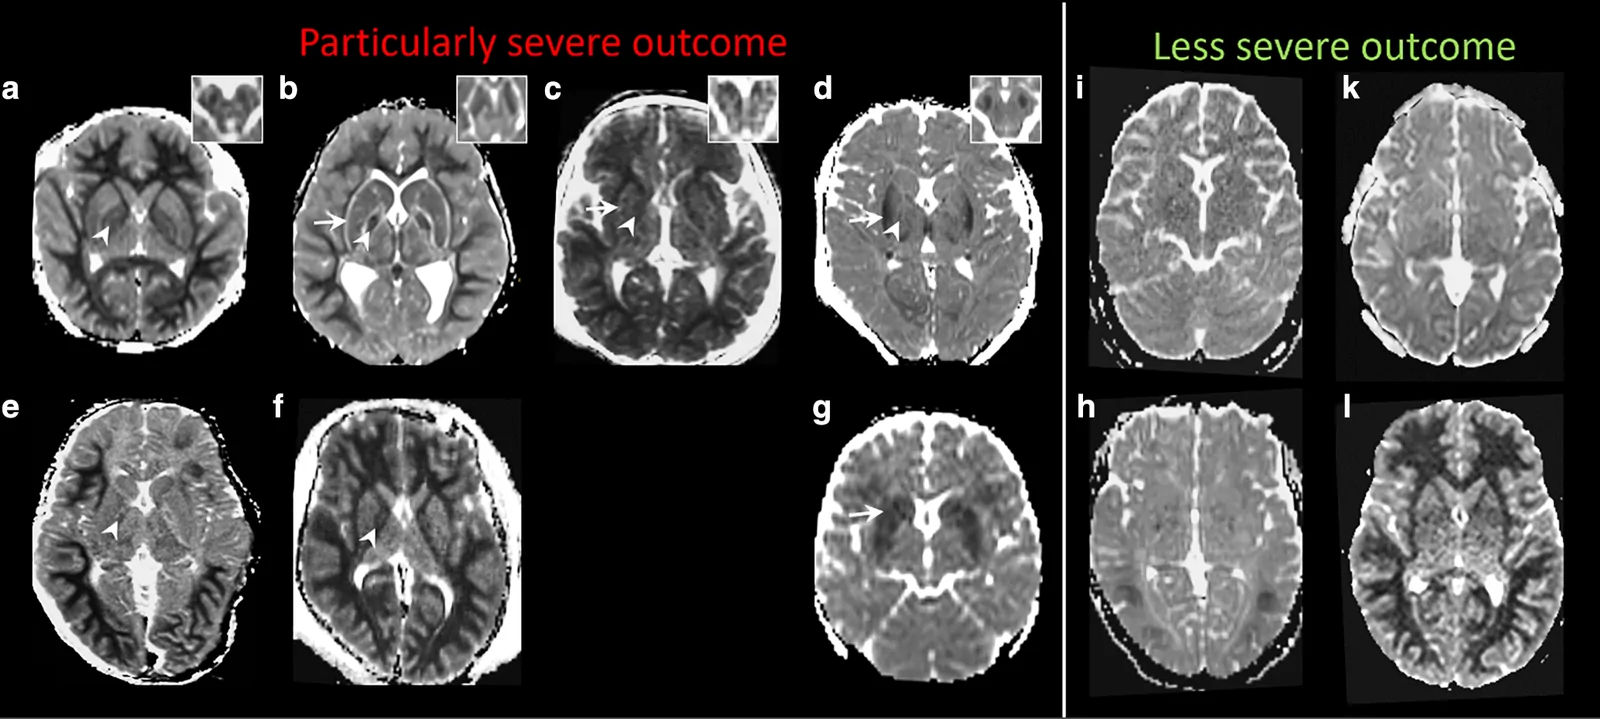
ADC-maps obtained 4–5 days after the anoxic event. All 11 patients who received DWI/ADC MRI on days 4–5 after the anoxic event are represented by one axial image each. Particularly severe outcomes (seven patients; left) occurred in all patients showing restricted diffusion (dark on ADC) in any of the following deep gray matter structures: in the globus pallidus (6/7; a–f; arrowheads), in the caudate nucleus or putamen (4/7; b–d,g; arrows) and in the substantia nigra (4/7; a–d; inserts). In contrast, the four patients without restricted diffusion in any of these structures (right, h–l) all had less severe outcomes. Note the diffusion restrictions in the white matter in patients (a–c,e,f) and also in patients (h) and (l): In our data, this feature was not yet predictive during this time interval. Some days later, 6–9 days after the anoxic event, however, the *absence* of such signal alterations would predict less severe outcome (cp. Figure 3 and 4)
